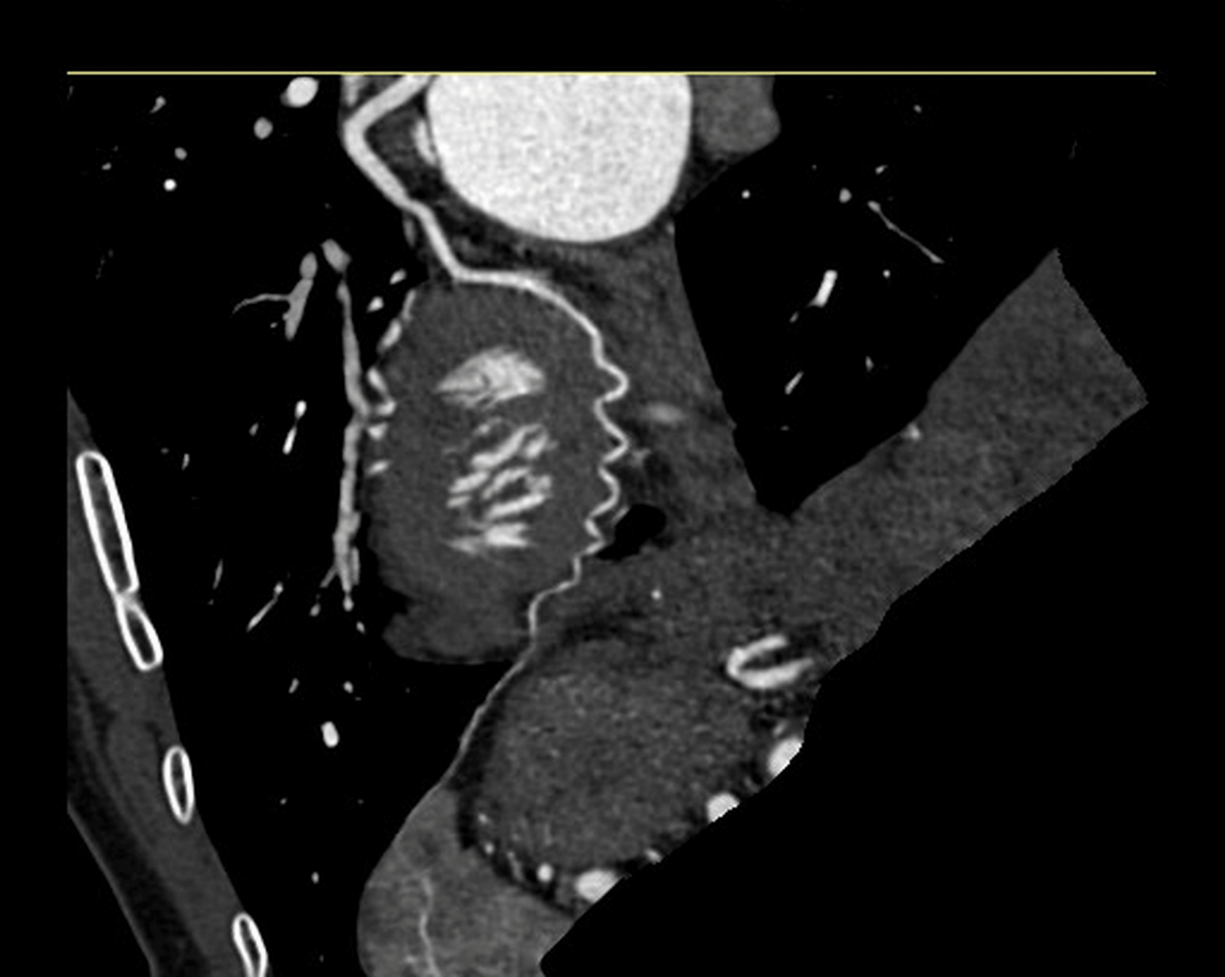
Follow-up CT coronary angiography of the LCx artery Curved planar reformatted CT coronary angiography at six-month follow-up demonstrated a patent LCx artery with interval resolution of the previously noted distal narrowing, consistent with spontaneous healing after conservative management of SCAD. LCx: left circumflex; SCAD: spontaneous coronary artery dissection
